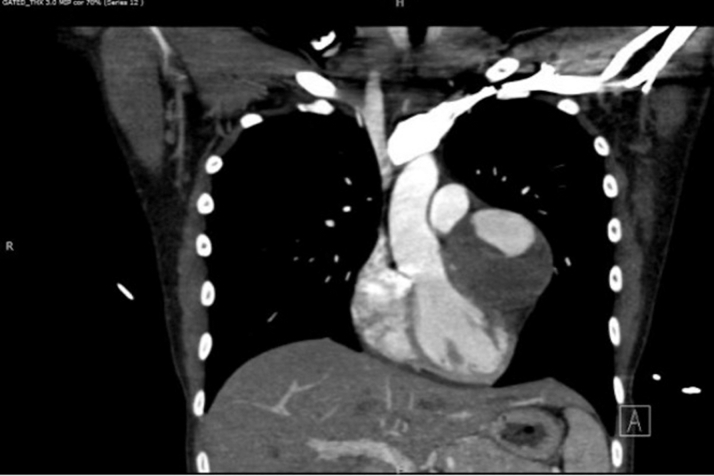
Computed Tomography Angiography Showing Proximal Left Anterior Descending Artery Aneurysm With Large Intramural Thrombus
